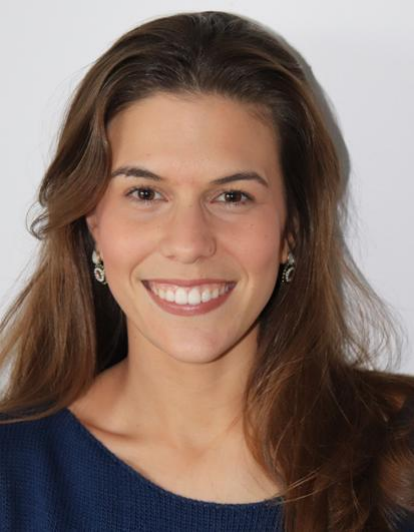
Rita Salgueiro Neto graduated in Medicine in 2016 from the Faculty of Medicine at the University of Lisbon and is currently completing her final year of residency in Obstetrics and Gynecology in Portugal. She has developed a strong interest in obstetric ultrasound and prenatal diagnosis, with a particular focus on optimizing maternal=E2=80=93fetal health through advanced imaging techniques. Beyond her clinical training, she is committed to research and academic work aimed at enhancing diagnostic precision and contributing to better outcomes in maternal and foetal medicine.
